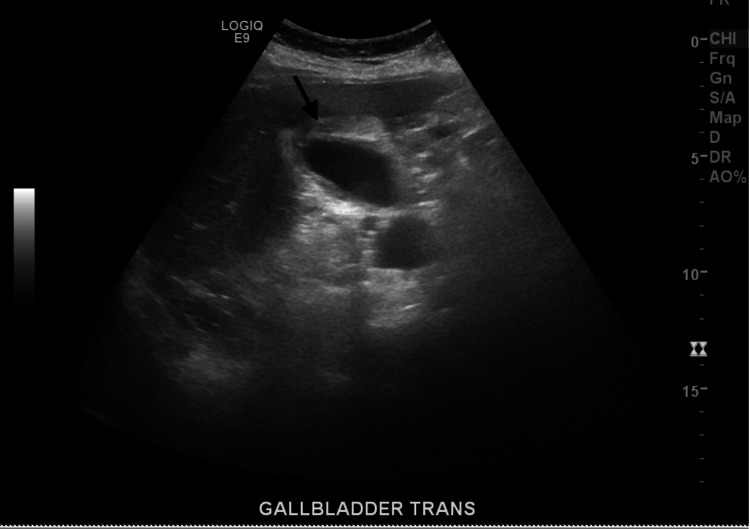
Ultrasound of gallbladder: arrow showing pericholecystic fluid collection
